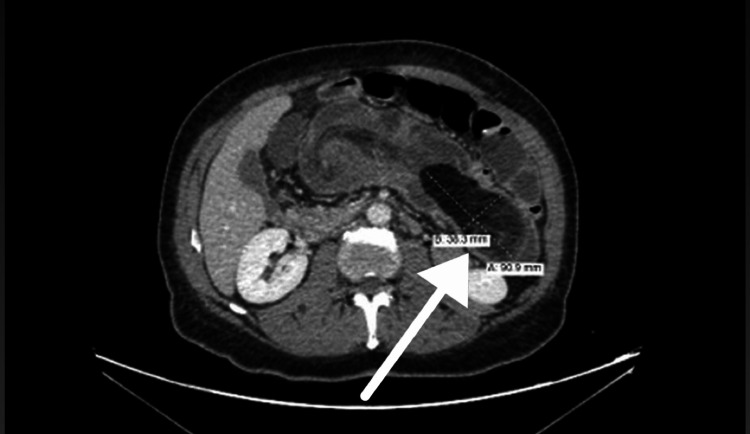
Axial contrast-enhanced CT scan of the abdomen showing a well-defined fat-density lesion in the left upper quadrant, embedded within the large bowel, measuring approximately 5 x 9 x 5.1 cm. The lesion is consistent with a colonic lipoma and serves as the lead point for the intussusception. A red arrow highlights the lipoma
